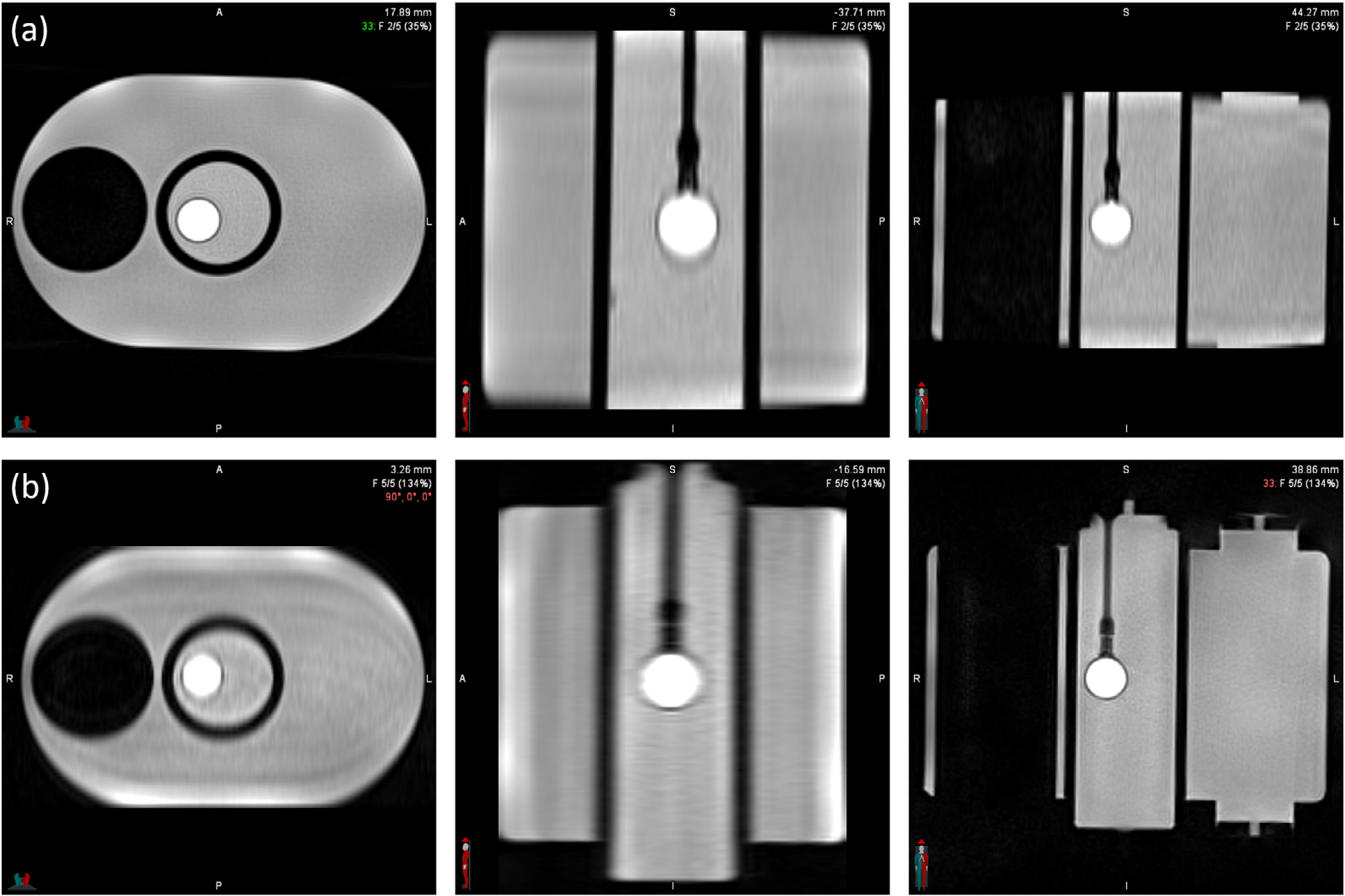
Corresponding axial, sagittal, and coronal views of an MR image for (a) axial and (b) coronal acquisition.
